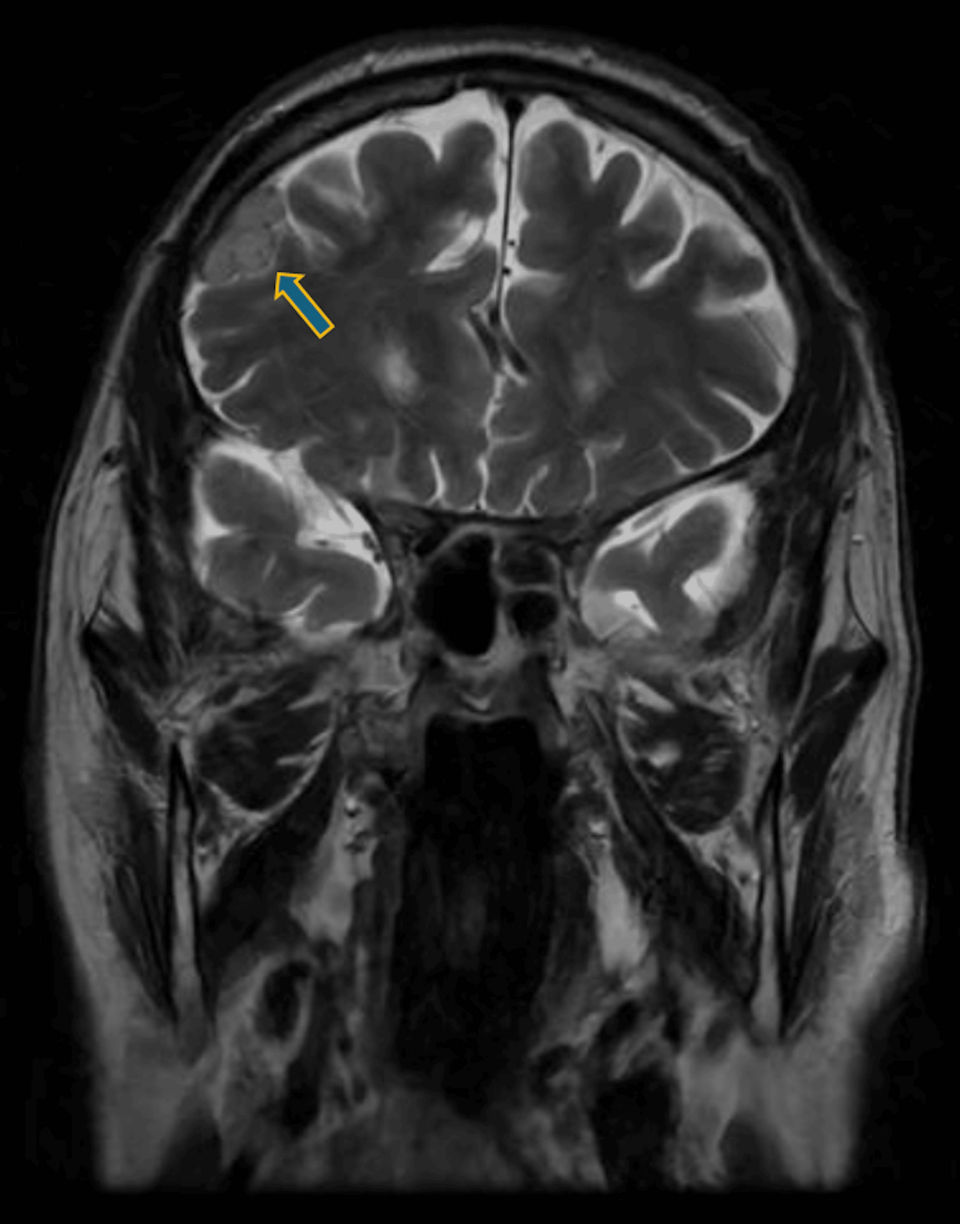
Brain MRI (T2 coronal) without contrast showed a right frontal lobe meningioma.
